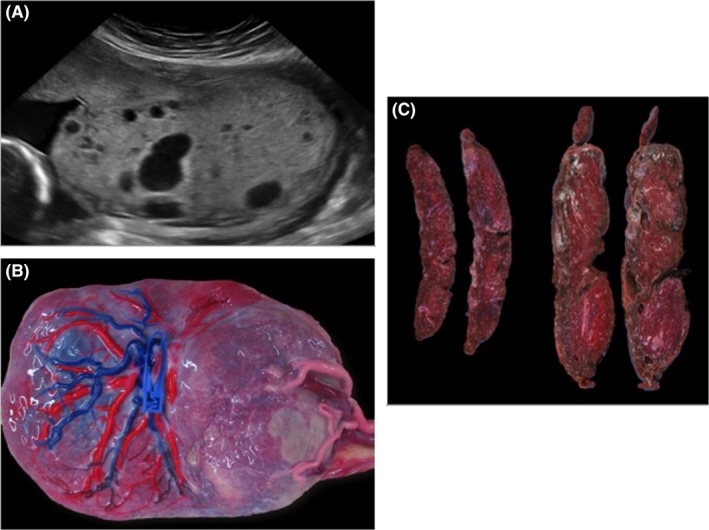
A, 2‐D ultrasound image demonstrating normal and molar aspect of the placenta of twin 1 (left) and twin 2 (right), respectively. B, Placenta after color dye injection showing the absence of vascular anastomoses. Twin 1 has an eccentric cord insertion (1 clamp‐left), whereas twin 2 has a marginal cord insertion (right). C, Macroscopic images demonstrating the normal placental tissue of twin 1 (left) and the cystic enlarged part belonging to twin (right)
